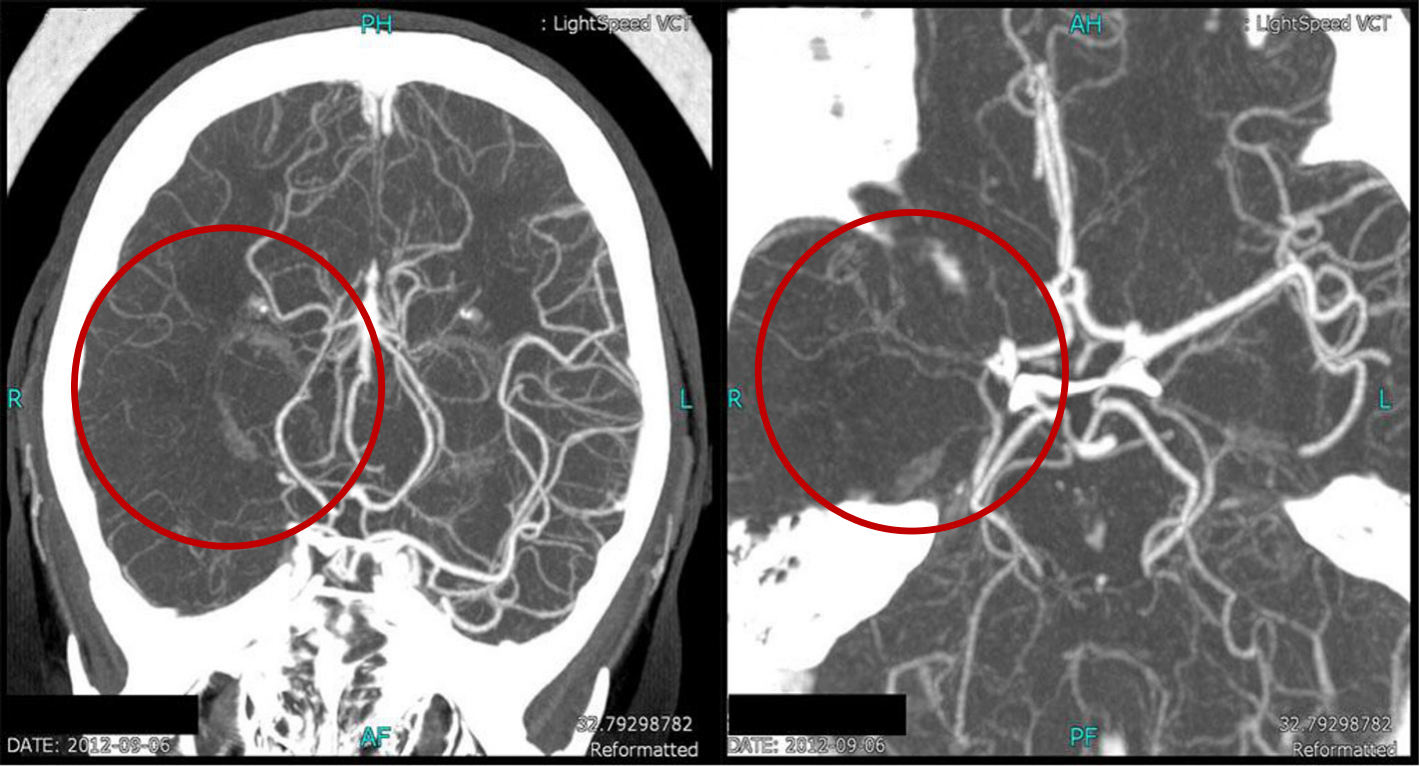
Head computed tomographic angiography (CTA) showing absence of the middle cerebral artery (MCA) on the right side (red circle).
